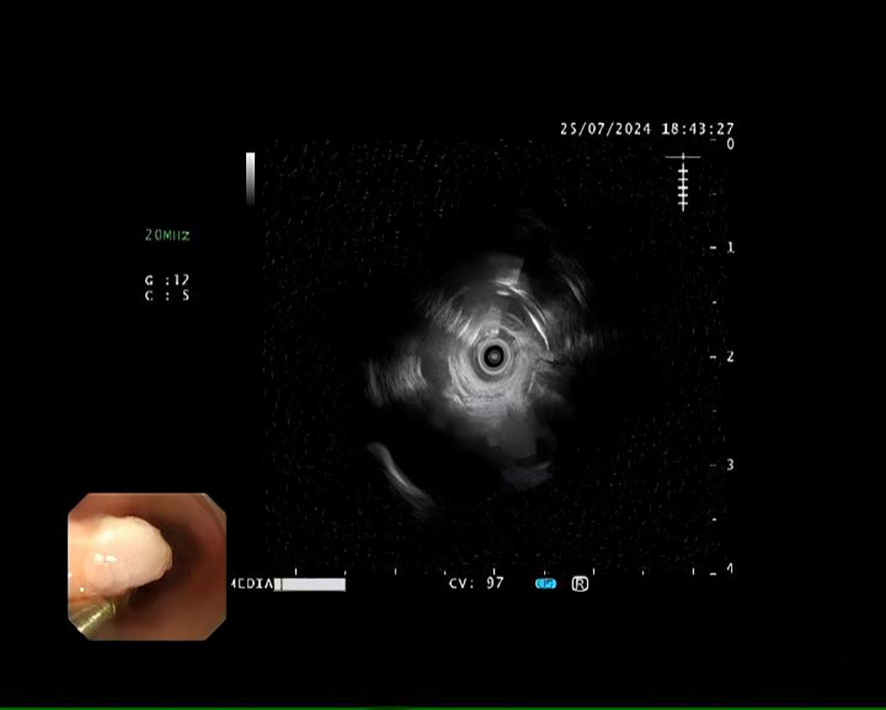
Hypoechoic tumor measuring approximately 12 mm in diameter, confined to the muscularis mucosae, with a smooth contour and well-defined borders, as seen on endoscopic ultrasonography.
